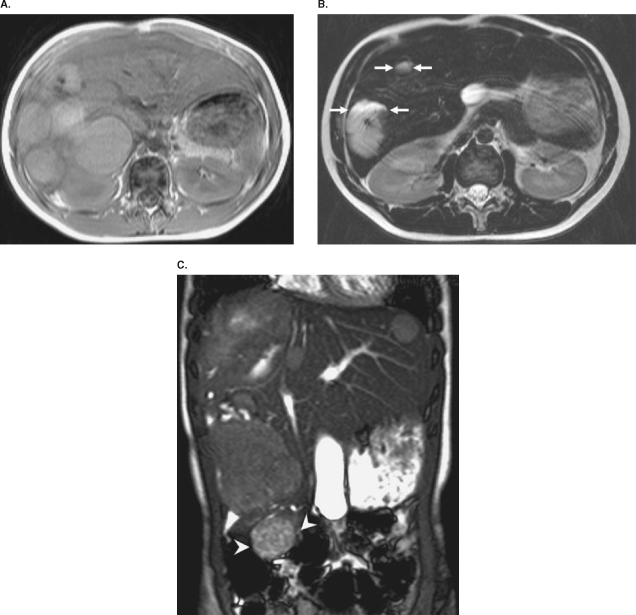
Non-contrast T1-weighted axial magnetic resonance (MR) image (A) shows multiple well defined circumscribed hyperintense masses. On T2-weighted axial MR image (B), lesions demonstrate high signal intensity changes. Two lesions also reveal fluid-fluid level (arrows). On coronal T2-weighted turbo-spin echo MR image (C) one right lobe mass extends outside the liver contours (arrowheads).
